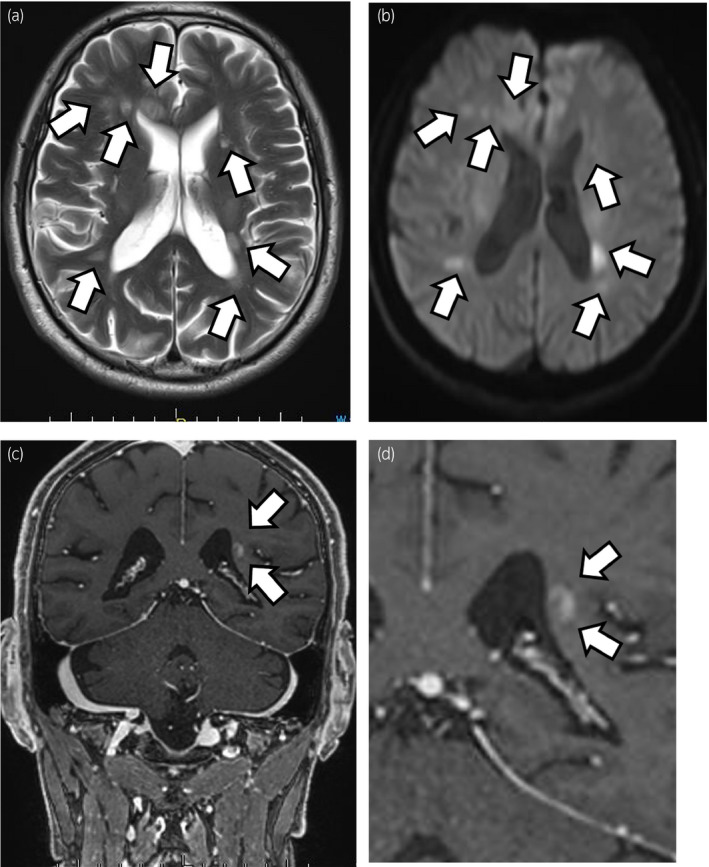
Brain MRI shows high signal in T2‐weighted images and diffusion‐weighted images in the cerebral white matter (arrows: CNS demyelination). (a) T2‐weighted images; (b) diffusion‐weighted images; (c) brain‐enhanced MRI shows an open‐ring sign; (d) enlarged image of panel c.
